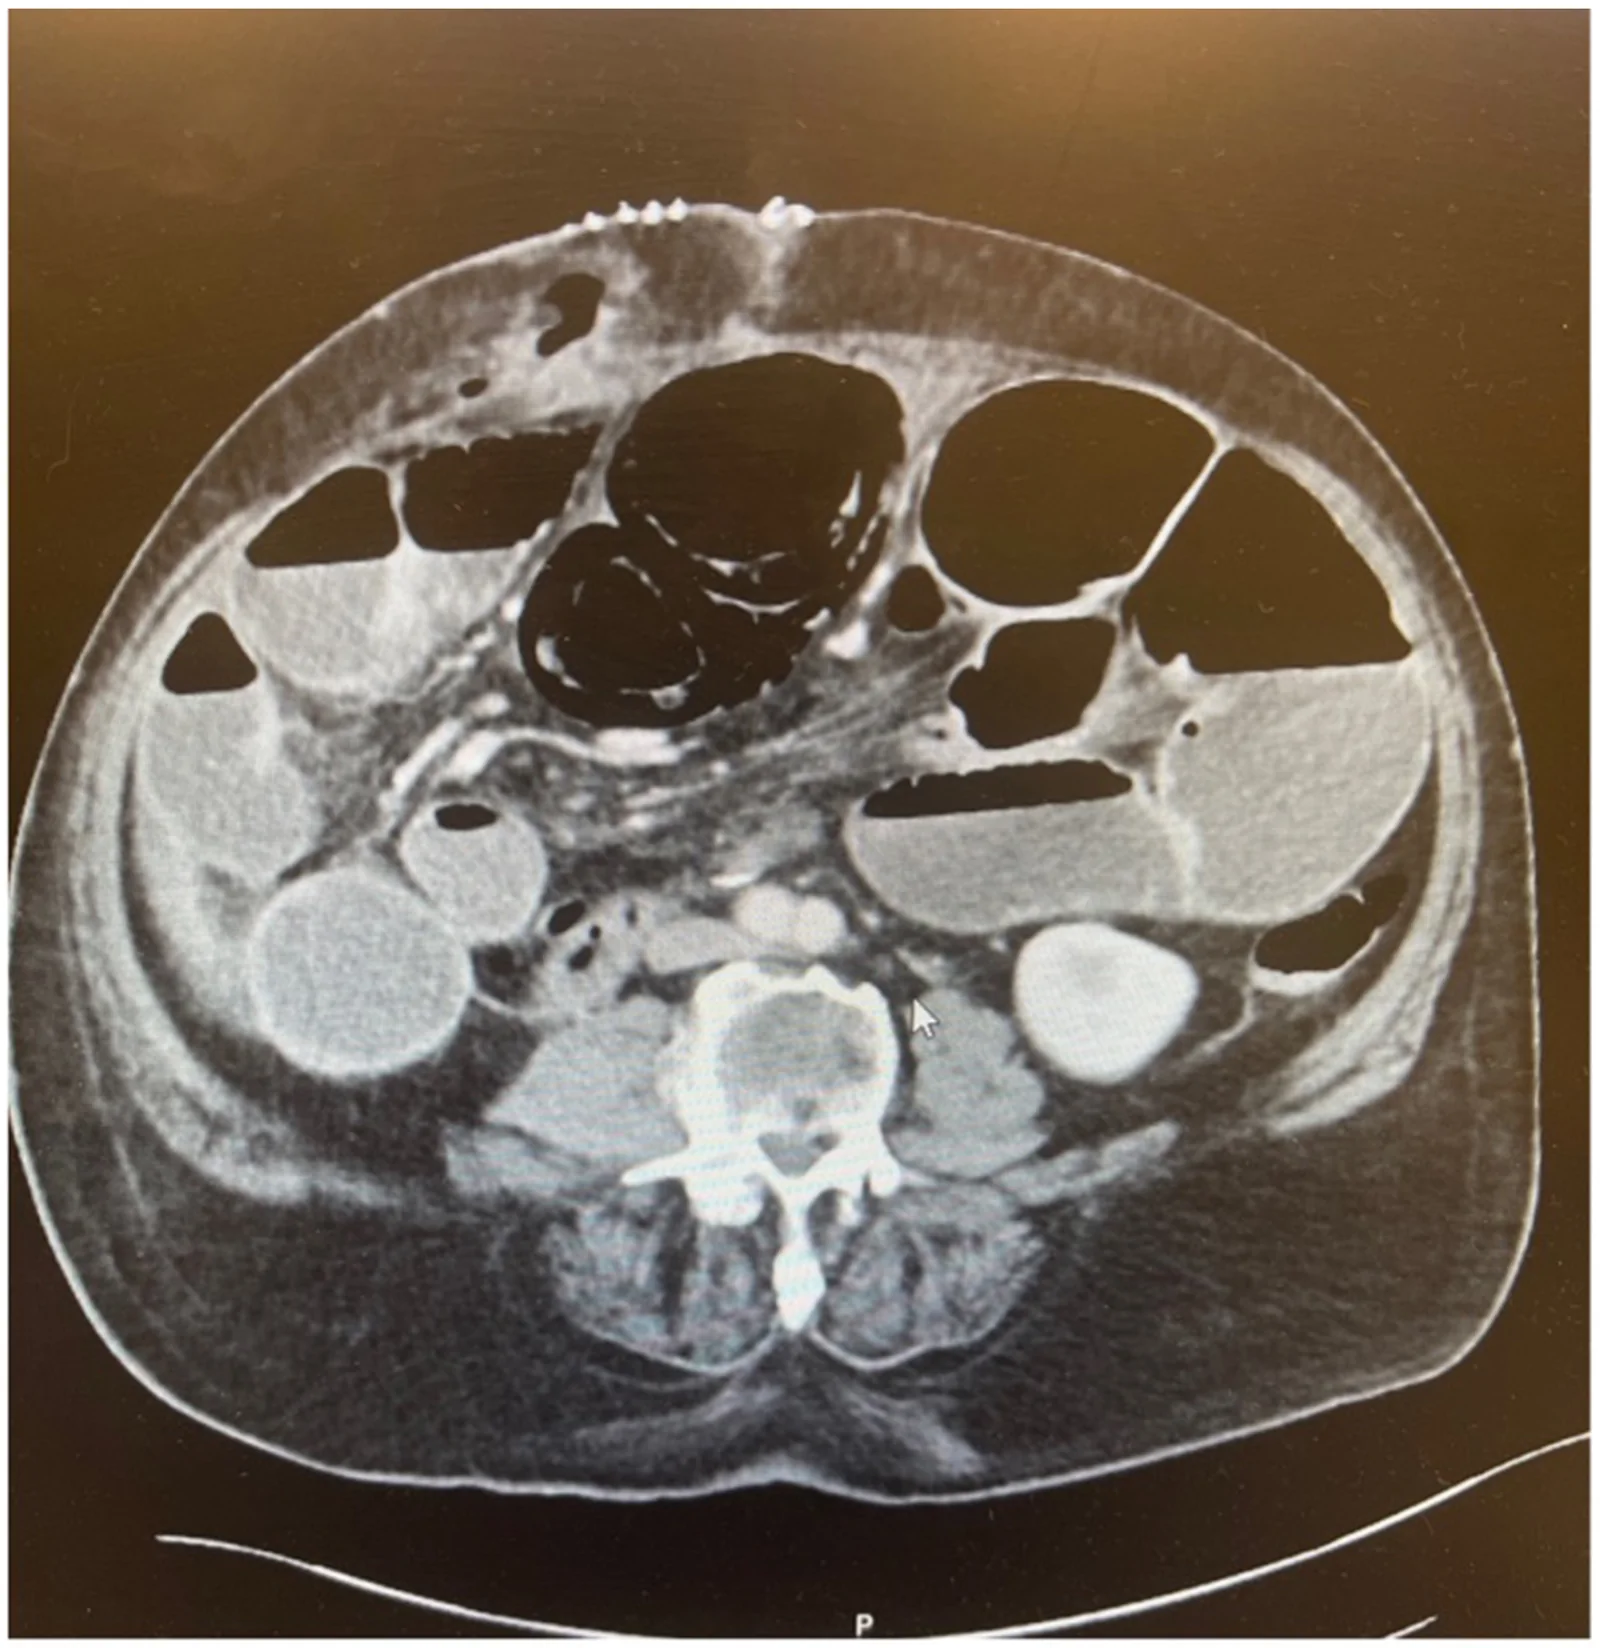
Axial CT image (POD eight) showing improvement in PI following conservative management. Compared with Figure 1, the bowel wall demonstrates preserved enhancement and a marked reduction in intramural gas. There is continued bowel dilation, but no evidence of free intra-abdominal air or portal venous gas. CT = computed tomography; PI = pneumatosis intestinalis; POD = postoperative day
